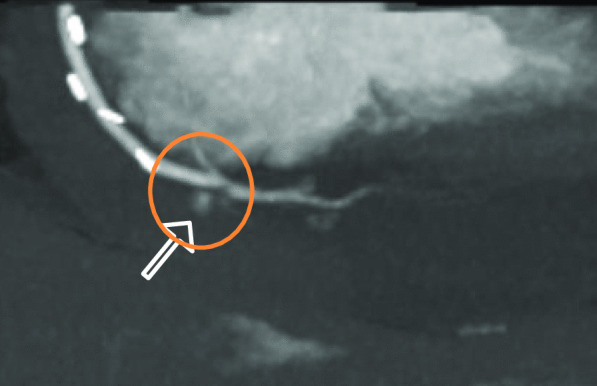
Active extravasation of contrast agent on abdominal CT Scan, arterial phase, paracoronal plane with MIP- reconstruction. (CT: computer tomography; MIP: maximum intensity projection)
